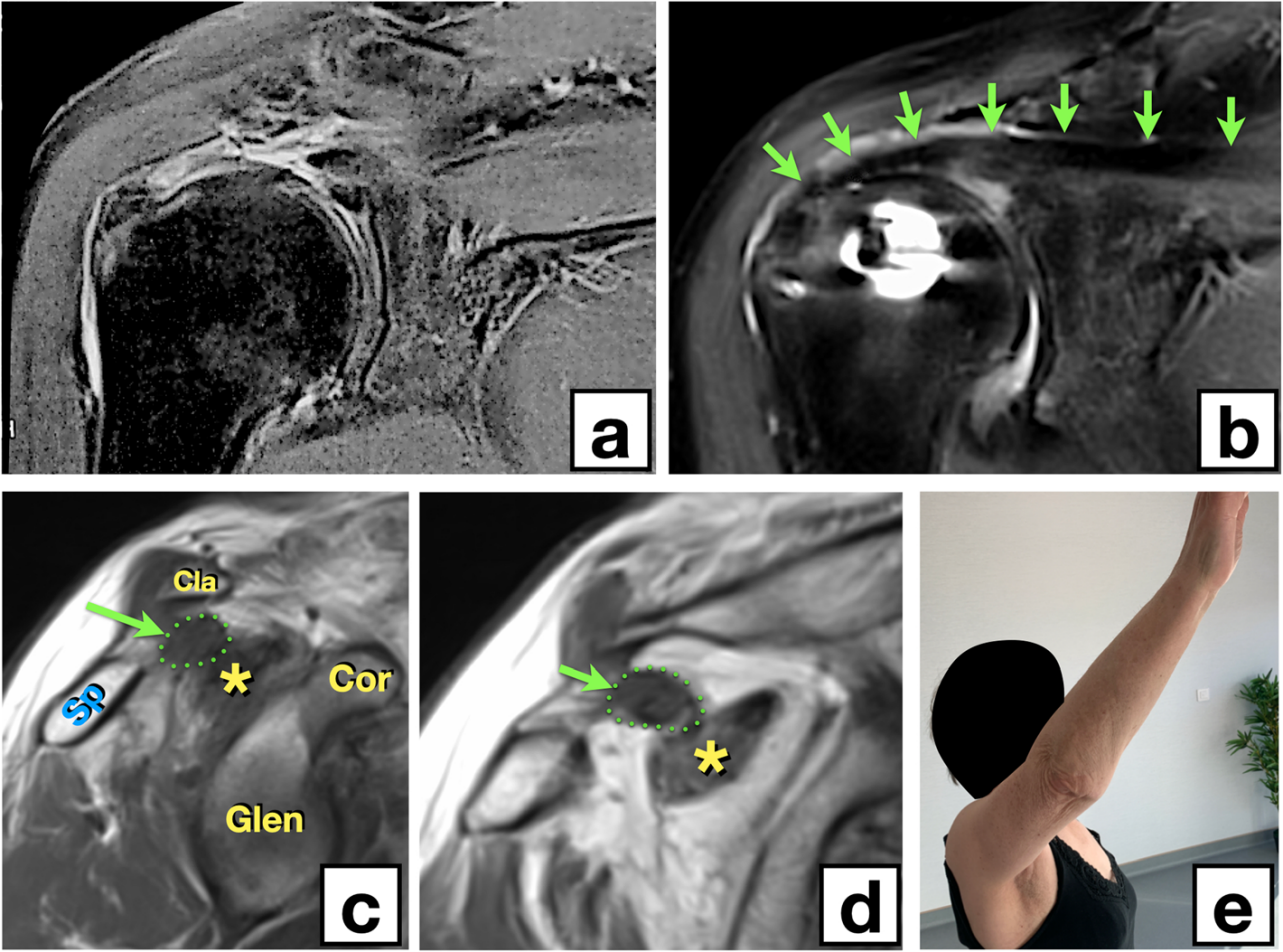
Clinical case. a Preoperative MRI showing the fully retracted tear of the supraspinatus. b MRI at 1 year postoperatively showing the incorporated GRAST graft (green arrows) from the humerus to the supraspinatus fossa. c, d Sagittal sections showing the GRAST graft in the supraspinatus fossa (green dotted line). Asterisk: amyotrophic supraspinatus. Sp: Spine of the scapula. Cla: Clavicle. Cor: Coracoid. Glen: Glenoid of the scapula. e Anterior elevation of the arm
